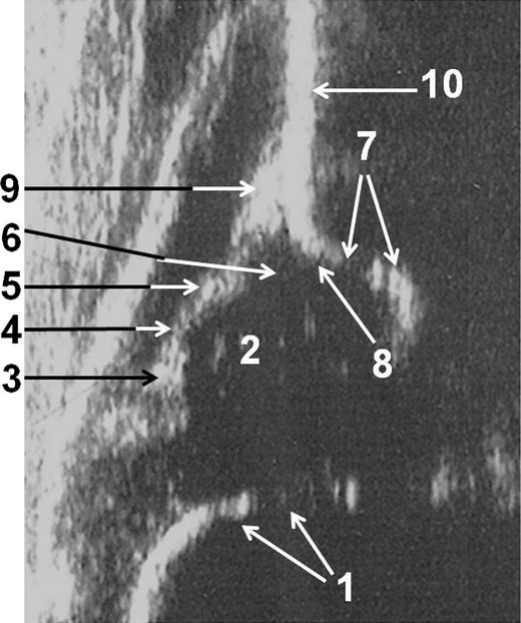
Anatomical identification of the structures in an infantile hip sonogram in the frontal plane at rest: *1* chondro-osseous junction, *2* femoral head, *3* synovial fold, *4* joint capsule, *5* acetabular labrum, *6* cartilage roof, *7* lower limb of the ilium and bony roof, *8* bony rim (the point where the concavity of the bony acetabular roof changes to the convexity of the iliac bone or the most lateral point of the acoustic shadow in the bony acetabular roof), *9* perichondrium, *10* iliac bone
